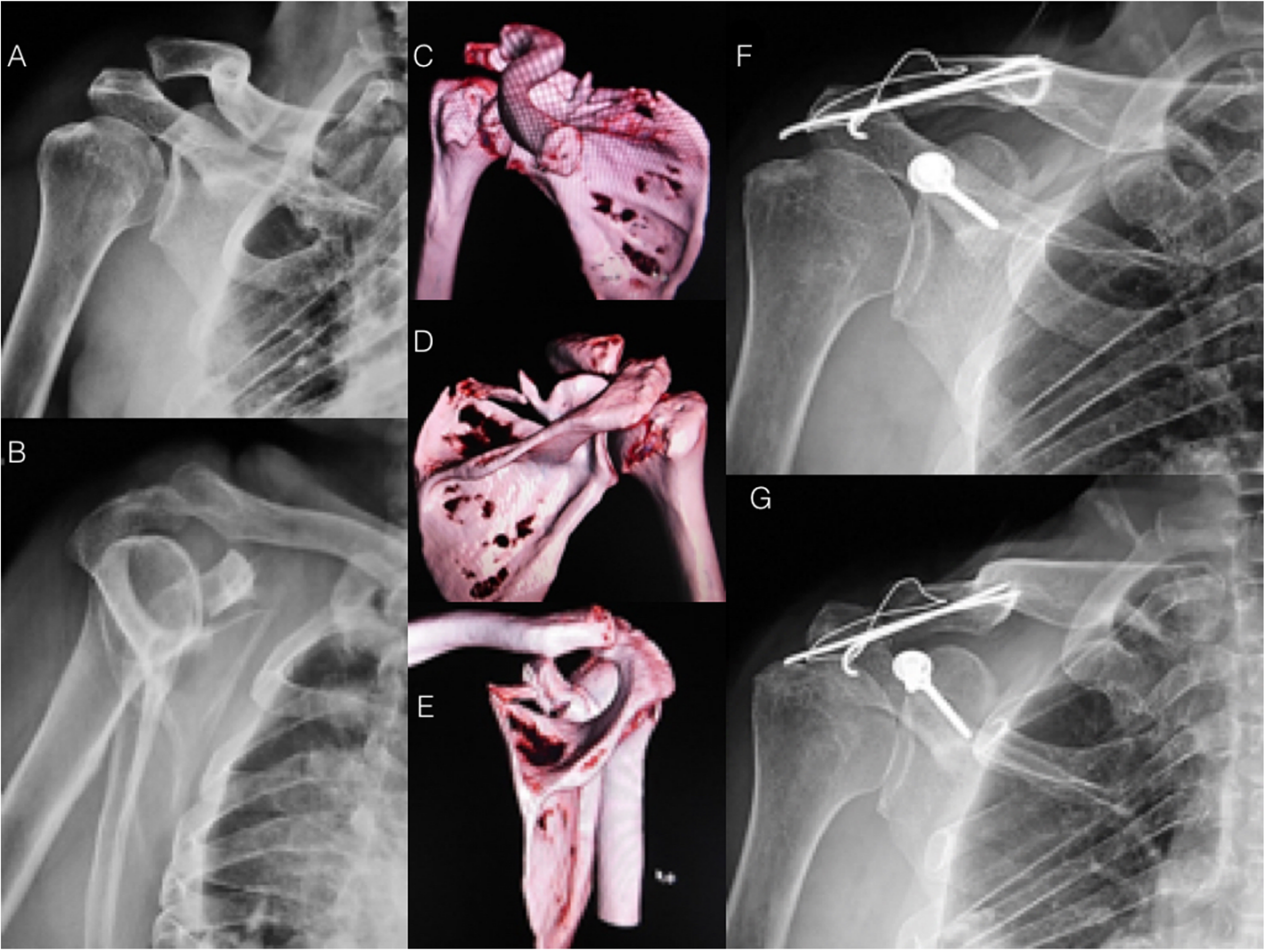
Radiographs (a and b) and 3D-CT reconstruction (c, d, and e) showing the fractures of the coracoid process and scapular spine associated with acromioclavicular dislocation. f and g: Postoperative radiographs showing the coracoid process fracture fixation with two 3.5 mm cortical screws and the acromioclavicular fixation with a static tension band
